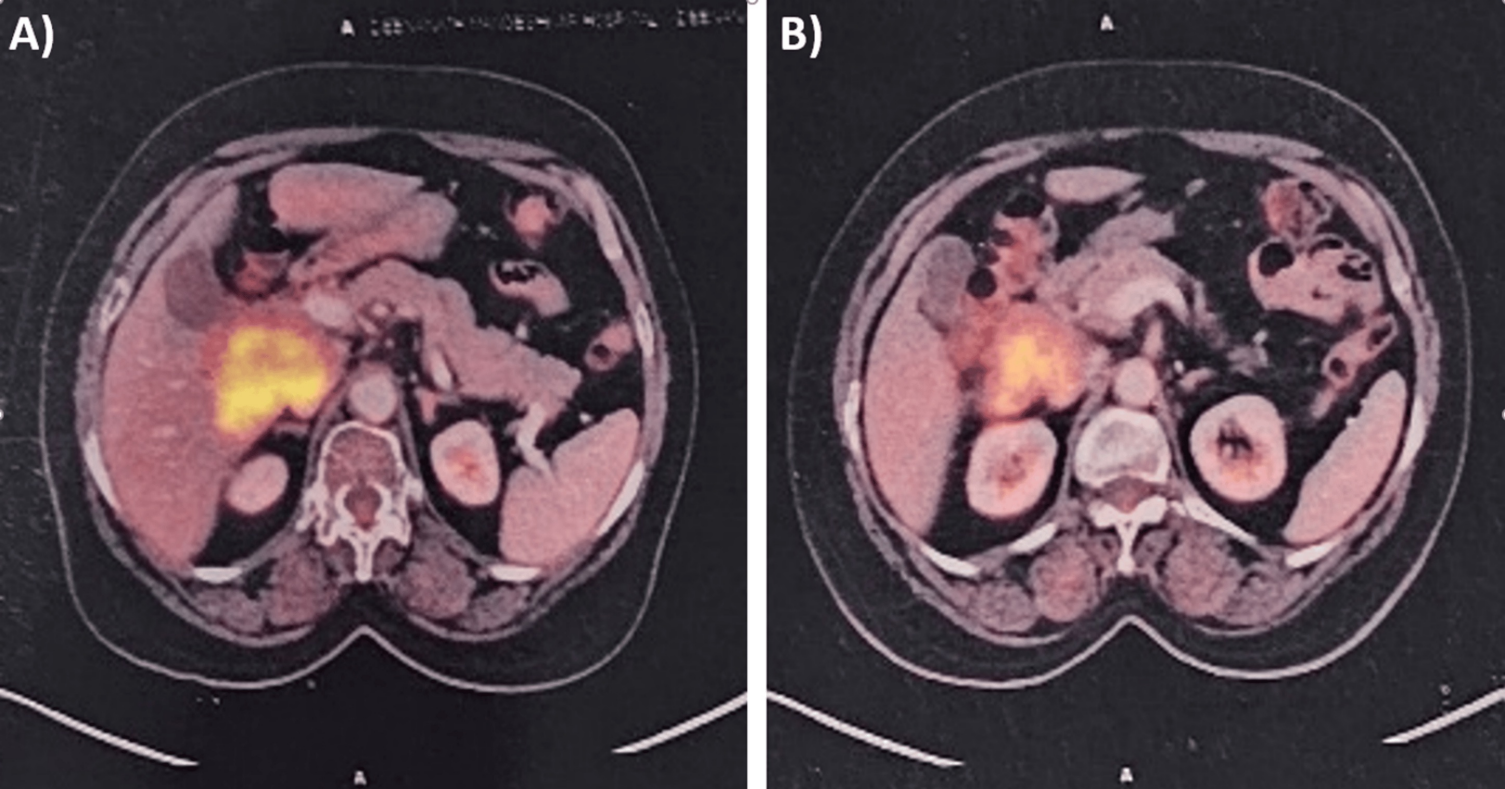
PET CT image (A) showing liver lesion with CBD encasement and (B) showing duodenal encasement PET: positron emission tomography; CT: computed tomography; CBD: common bile duct
